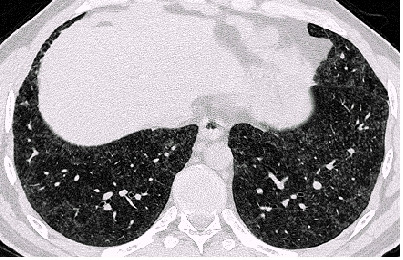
HRCT-scans. In the whole lung area there are small, ill-defined, diffuse opacities, in the upper right-hand field subpleural curvilinear lines. Figure 3 upper field, figure 4 middle field, Figures 5 and 6 lower field (case 10, table 5 and 6) [18]
